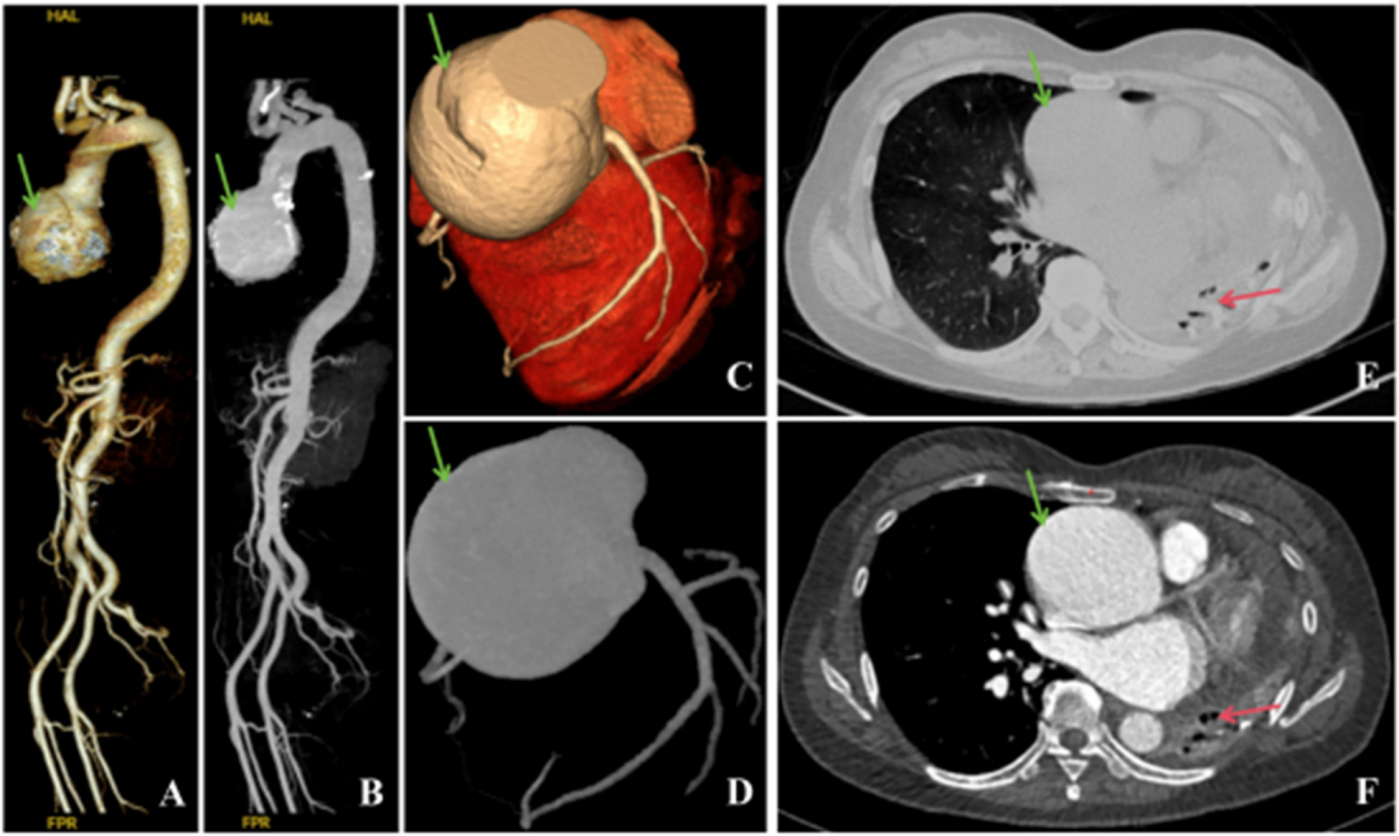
Preoperative thoracoabdominal aorta and coronary artery CTA, thoracic enhanced CT. (A,B) Ascending aortic aneurysmal dilation, tortuous course of the thoracic aorta (indicated by the green arrow), no abnormality observed in the abdominal aorta. (C,D) Coronary CTA: Ascending aortic aneurysmal dilation (indicated by the green arrow), myocardial bridge in the distal segment of the left anterior descending artery. (E,F) Aneurysmal dilation (indicated by the green arrow) with left lung destruction (indicated by the red arrow), secondary pulmonary tuberculosis in the right lung, a small amount of effusion in the left pleural cavity, and cardiac enlargement.
